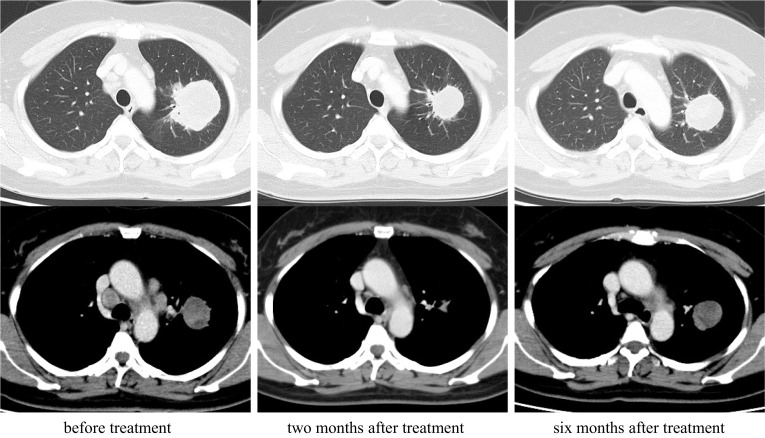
Computed tomography showed that the lung tumor had regrown, while the lymph nodes had shrunk after 6 months of the alectinib treatment
